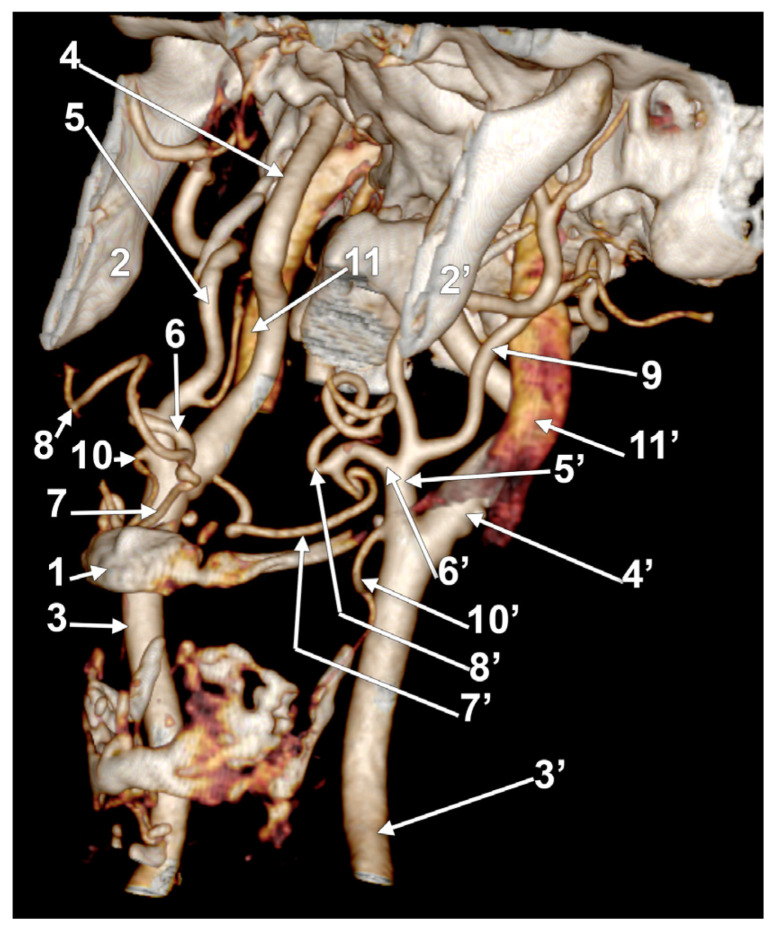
Bilateral linguofacial trunk. Computed tomography angiogram. Three-dimensional volume rendering. Left inferior view. 1. hyoid body; 2, 2’. gonial angles; 3, 3’. common carotid arteries; 4, 4’. internal carotid arteries; 5, 5’. external carotid arteries; 6, 6’. linguofacial trunks; 7, 7’. lingual arteries; 8, 8’. facial arteries; 9. left occipital artery; 10, 10’. superior thyroid arteries; and 11, 11’. internal jugular veins.
